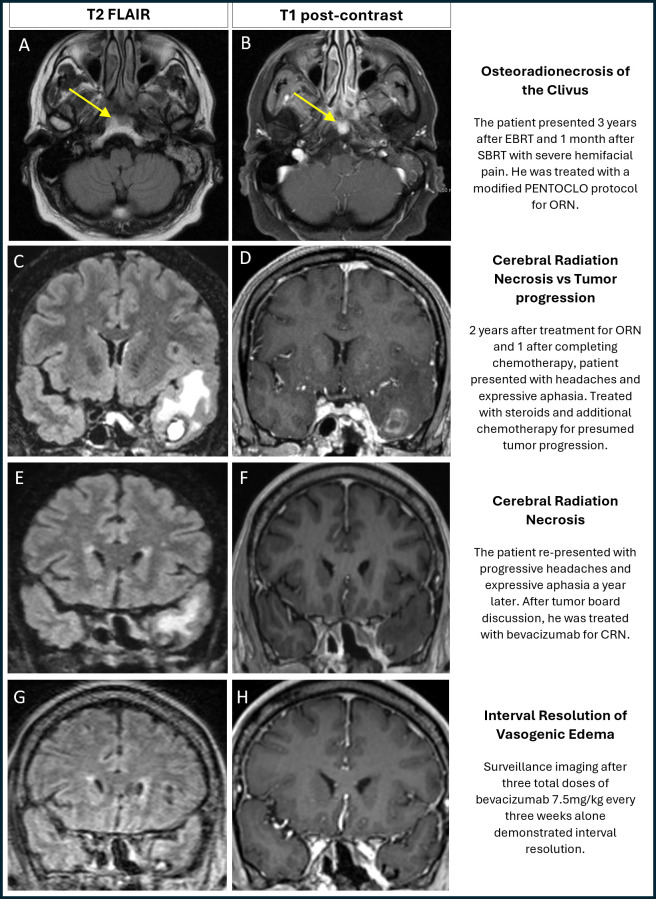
Neuro-imaging depicting osteoradionecrosis, delayed cerebral radiation necrosis, and treatment response. Our patient developed skull base osteoradionecrosis and late radiation necrosis of the temporal lobe, 4 years after treatment as seen in sequential MRI. Initial osteoradionecrosis of the clivus and posterior nasopharynx seen as hypointensity in axial T2 FLAIR sequence ((A), yellow arrow) and enhancement in axial T1 post-contrast [(B), yellow arrow]; patient treated with PENTOCLO protocol. 2 years after treatment for osteoradionecrosis, surveillance MRI showed a 1.6 x 1.3 cm hemorrhagic mass of the left temporal lobe tip with vasogenic edema in coronal T2 FLAIR sequence (C) and enhancement in coronal T1 post contrast (D), as well as changes concerning for nasopharyngeal carcinoma progression on the left aspect of nasopharynx and left posterior nasal cavity with tumor involving left pterygopalatine fossa, left skull base, posterior orbit, and left cavernous sinus; treated as tumor progression. Almost two years later patient continued to have symptoms of headache and expressive aphasia, and 0.6 cm and 0.5 cm similar-appearing lesions in the parenchyma of the left temporal lobe with associated vasogenic edema were seen in coronal T2 FLAIR (E) and coronal T1 post contrast (F) MRI sequences. Nasopharyngeal carcinoma demonstrated stable interval changes, increasing suspicion for CRN. After treatment with bevacizumab, interval resolution of vasogenic edema consistent with response of radiation necrosis was observed in coronal T2 FLAIR (G) and coronal T1 post contrast (H). EBRT- external beam radiation therapy; SBRT- stereotactic beam radiation therapy; MRI- Magnetic resonance imaging; FLAIR- Fluid-attenuated inversion recovery; ORN- osteoradionecrosis; PENTOCLO protocol- One month of prednisone, amoxicillin/clavulanate, ciprofloxacin, and fluconazole followed by one month of pentoxifylline, alpha-tocopherol, prednisone, ciprofloxacin, and alendronate; CRN- Cerebral radiation necrosis.
